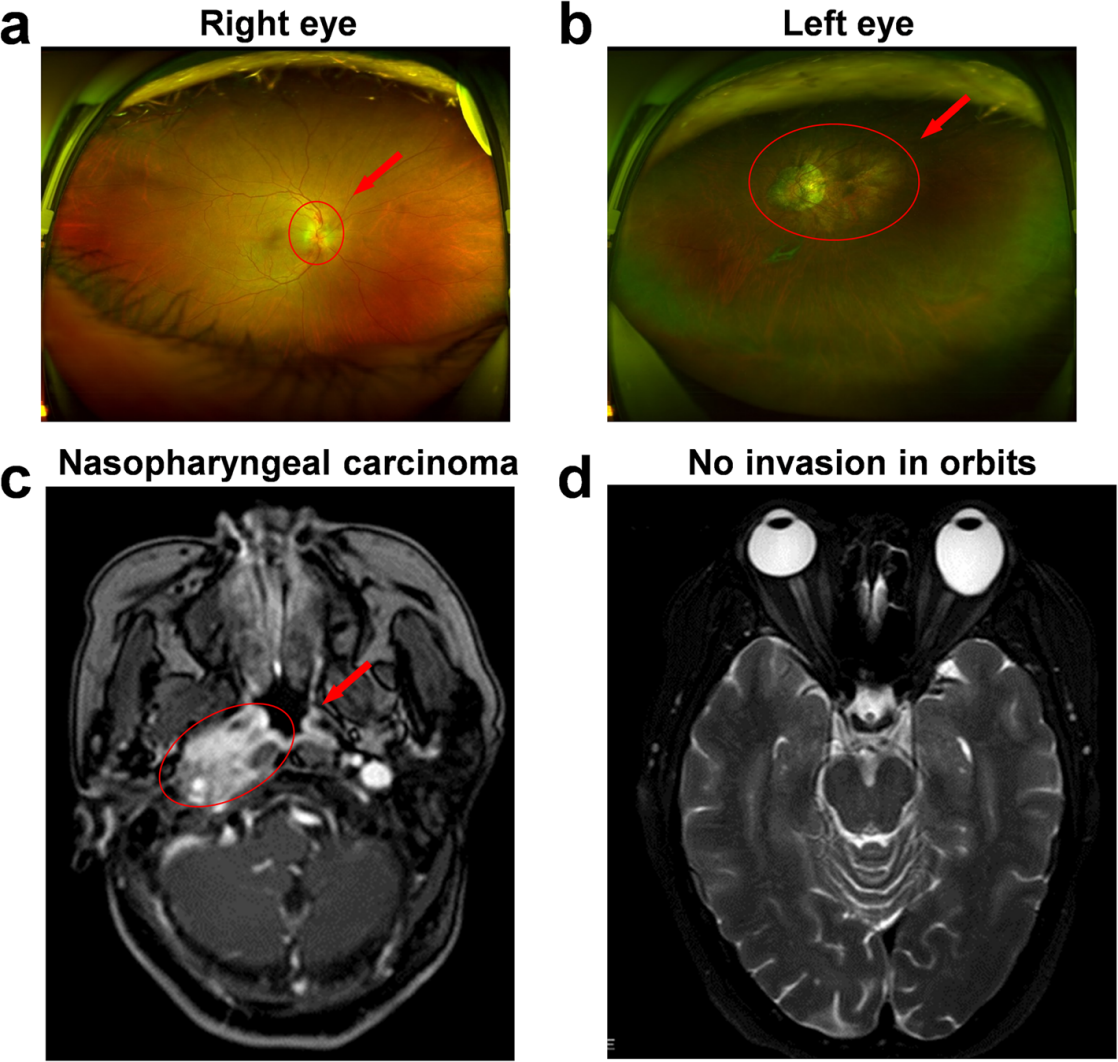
Ophthalmoscope examination and MRI images of orbits during chemotherapy. a: fundus photo of the right eye, b: fundus photo of the left eye (December 6th, 2017), c: MRI images of skull base invasion in nasopharyngeal carcinoma, d: MRI images of orbits (December 26th, 2017)
